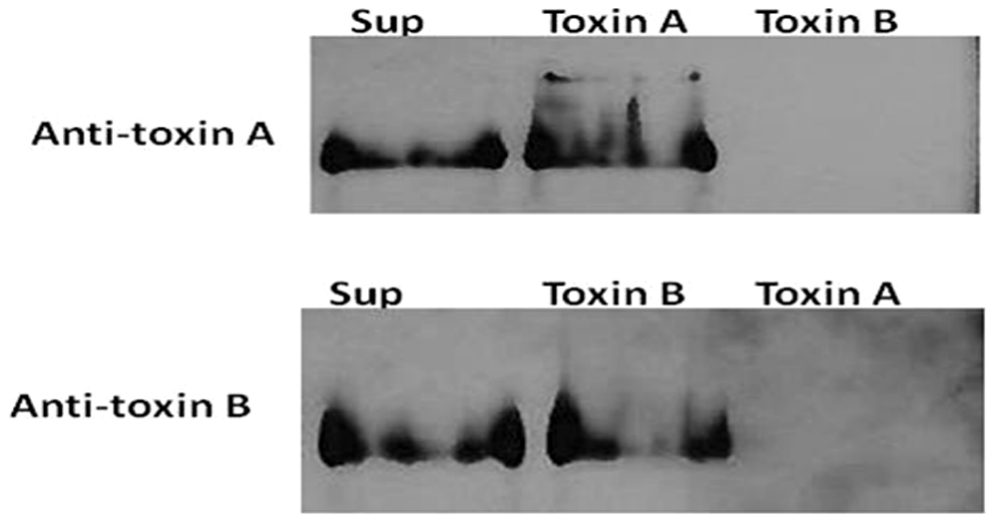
Immunoblot analysis of purified *C. difficile* toxins A and B. Purified proteins (80 µg each) were subjected to 6% PAGE and transferred onto PVDF membranes. Each membrane was probed using monoclonal primary antibodies specific for toxin A or B. The Pierce ECL Western Blotting Kit was used to detect the bound antibodies. The membrane was exposed to X-ray film (Molecular Technologies, St Louis, MO) and processed using a Konica film processor (Konica Corporation, Tokyo, Japan). Sup, crude culture supernatant; Toxin A, purified toxin A; Toxin B, purified toxin B.
